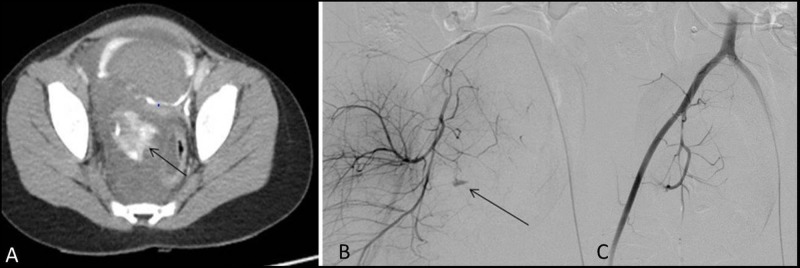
Child presenting with perineal injury due to glass A: Large pelvis hematoma with active extravasation (arrow) seen on CT. Bladder shows filling defect due to clot. B: Extravasation from branches of internal iliac artery (arrow). C: Successful embolization of abnormal vessel with no further extravasation CT: computed tomography
